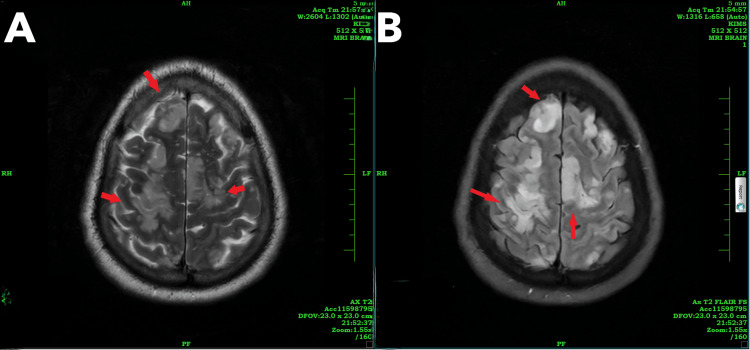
(A) T2-weighted MRI brain and (B) FLAIR sequence The red arrows highlight hyperintensities in the bilateral frontal cerebral hemispheres.
